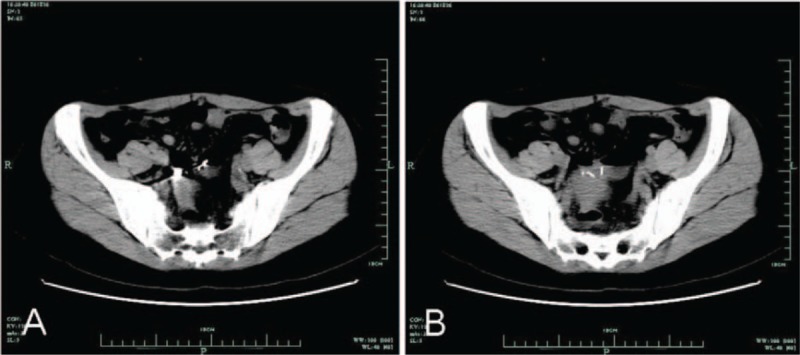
(A, B) Computed tomography reveals an IUD translocated to the sigmoid colon.
